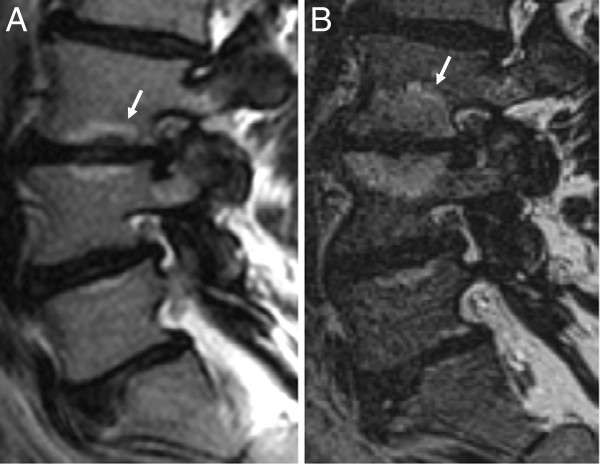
Increased size of Modic changes. Initial (A) and follow-up (B) sagittal T2-weigthed magnetic resonance images of one patient. Modic changes at L3/L4 increase in craniocaudal extent from <1/4 to >1/2 of the vertebral body height from image A to image B (arrows).
